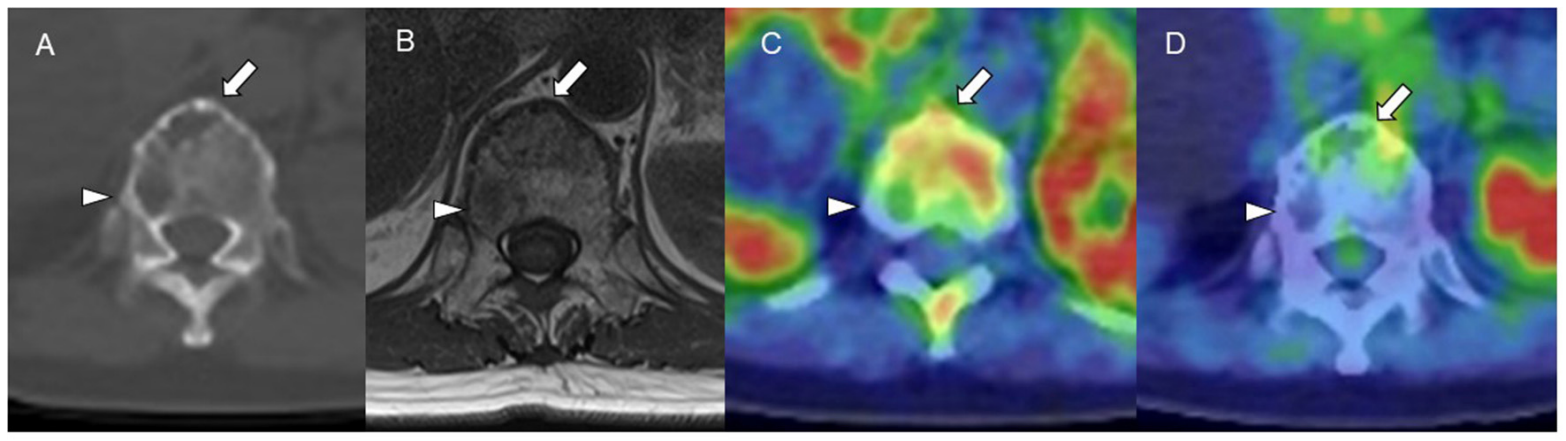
(A) CT (bone window). (B) MRI (TI-weighted image). (C) Carbon-11-labeled 4′-thiothymidine positron emission tomography/computed tomography (^11^C-4DST PET/CT). (D) 2-[^18^F]-fluoro-2-deoxy-glucose (^18^F-FDG) PET/CT. ^11^C-4DST could distinguish active (*arrow*) and inactive (*arrowhead*) myeloma lesions in a vertebral body, those that were regarded as all positive on MRI and CT and all negative on ^18^F-FDG.
